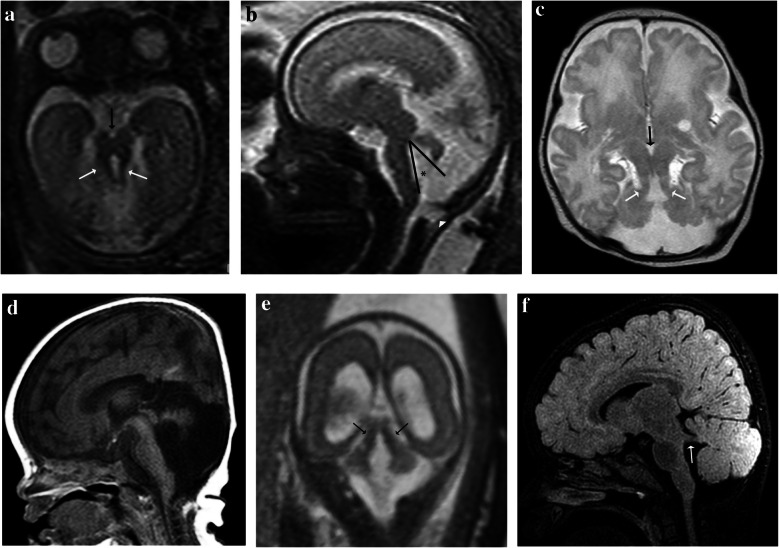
Joubert syndrome. Fetal MRI T2 SSFSE images at 23 weeks gestational age are notable for (a) the molar tooth sign in the axial plane, created by a deep interpeduncular cistern (*black arrow*) and thickened, horizontally oriented superior cerebellar peduncles (*white arrows*). The midline sagittal view (b) shows diffuse vermian hypoplasia (as opposed to an inferior-predominant vermian hypoplasia that is more typical of DWM) and a widened tegmento-vermian angle (*asterisk*), plus a suboccipital meningocele (*white arrowhead*). Postnatal brain MRI (c) axial T2 and (d) sagittal T1 redemonstrate these findings of Joubert syndrome. e A different fetal MRI T2 SSFSE coronal image at 22 weeks gestation shows a thickened appearance of the superior cerebellar peduncles (*black arrows*). f Postnatal brain MRI at 18 months of age demonstrates thickened and horizontally oriented superior cerebellar peduncles when viewed in a paramedian sagittal plane (*white arrow*)
